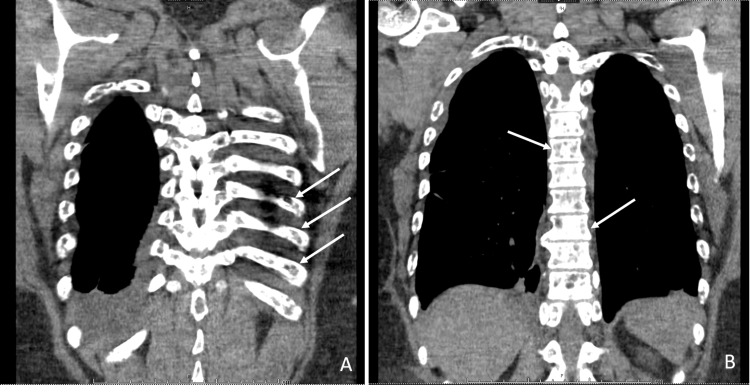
CT chest. CT chest showing diffuse lytic bone lesions in the ribs (A) and spinal column (B), with no evidence of a right hilar mass. CT: computed tomography
